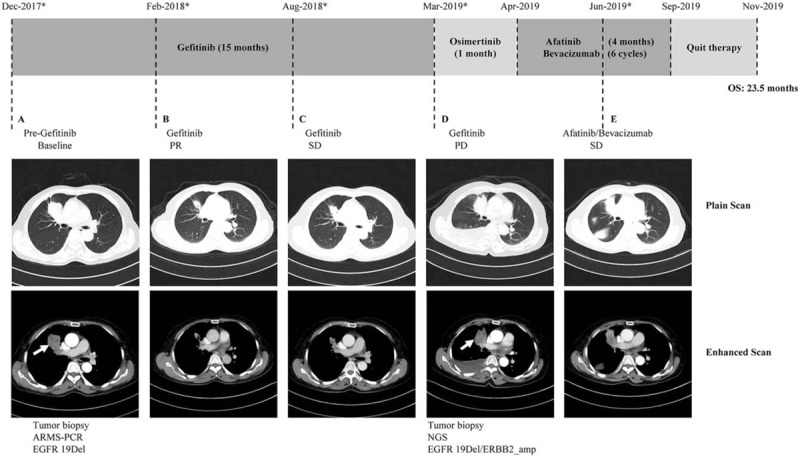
A clinical summary of the patient's treatment process. Upper panel shows the treatments for the patient with *EGFR*-mutated non-small cell lung cancer. Bottom panel shows computed tomography (CT) plain and enhanced scans of the patient's lung lesions before gefitinib therapy (A), partial response (PR) to gefitinib (B), stable disease (SD) on gefitinib (C), progressive disease (PD) to gefitinib (D), and SD on afatinib and bevacizumab (E). Genetic testing results of the biopsy tissues are shown below the corresponding CT images. The white arrow represents the position of sampling. ^∗^indicate the time points for response assessment.
